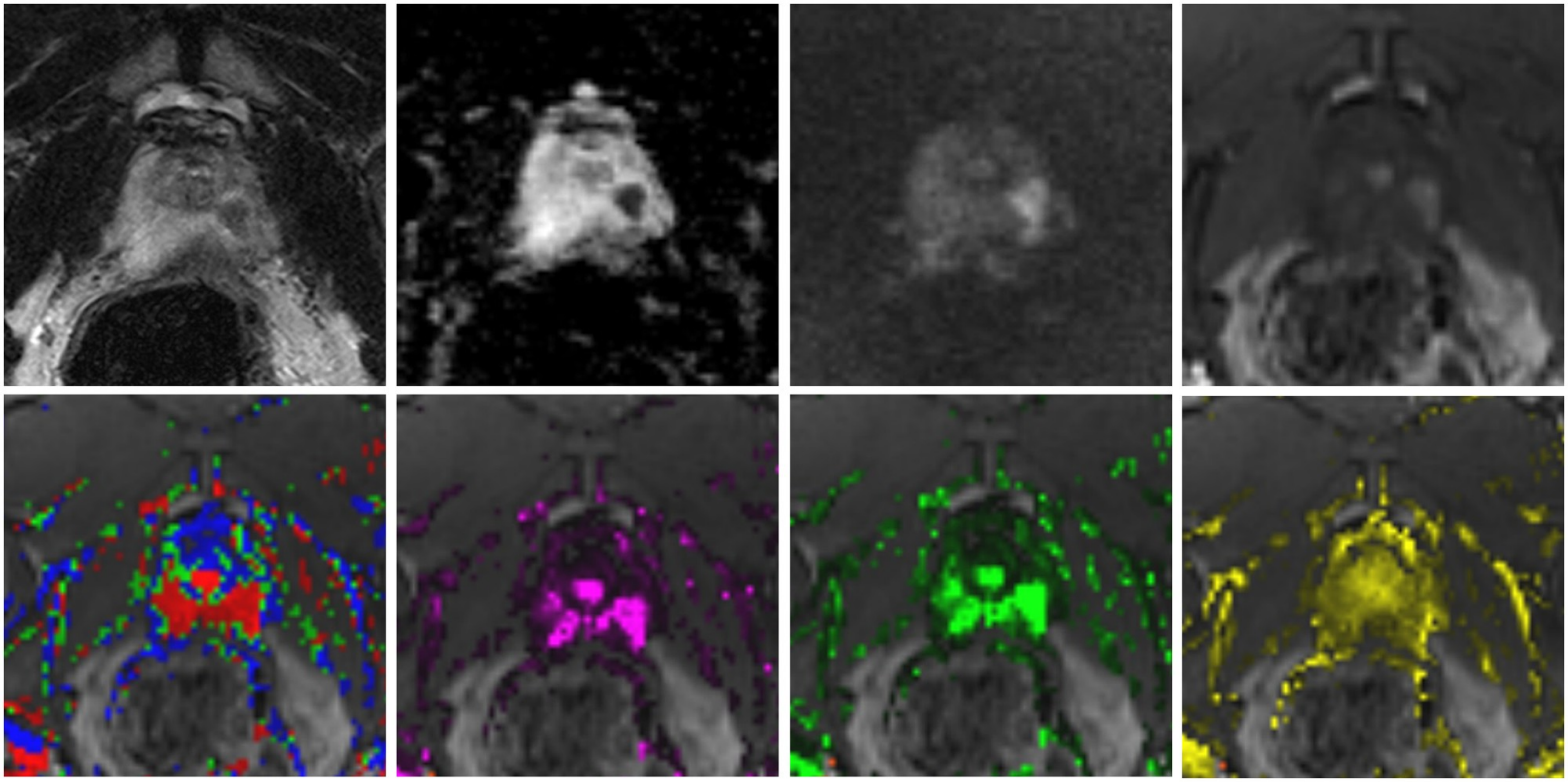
Peripheral PCa lesion is declinable in most of the perfusion maps. Example of a peripheral PCa lesion. *Upper row*: multiparametric MRI (from left to right with T2, DWI, ADC, and DCE showing a left peripheral carcinoma with gleason score 4+3 = 7 in a 48 year old patient. *Lower row*: corresponding perfusion maps (from left to right) colour-coded, K^trans^, k_ep_, and v_e_.
